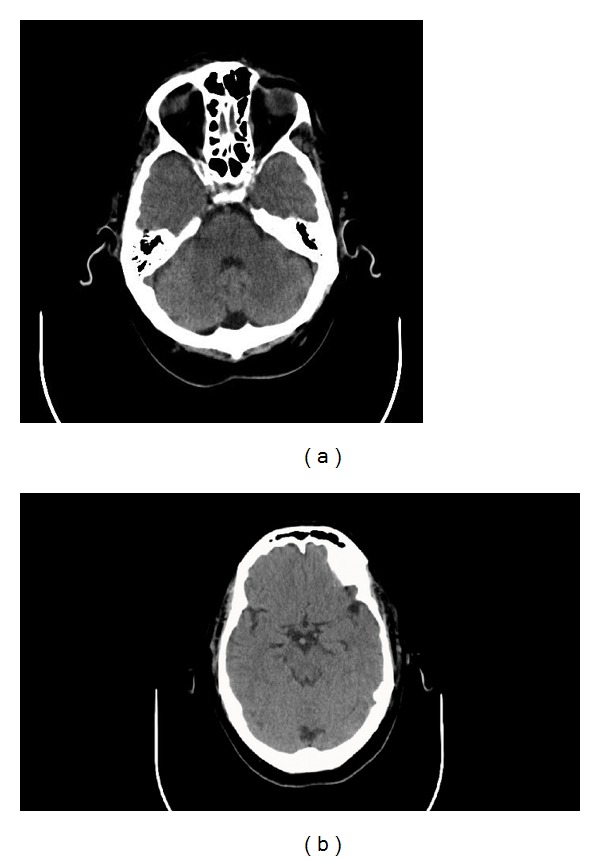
CT head scan on postoperative day 2 showed resolution of the pneumocephalus.
